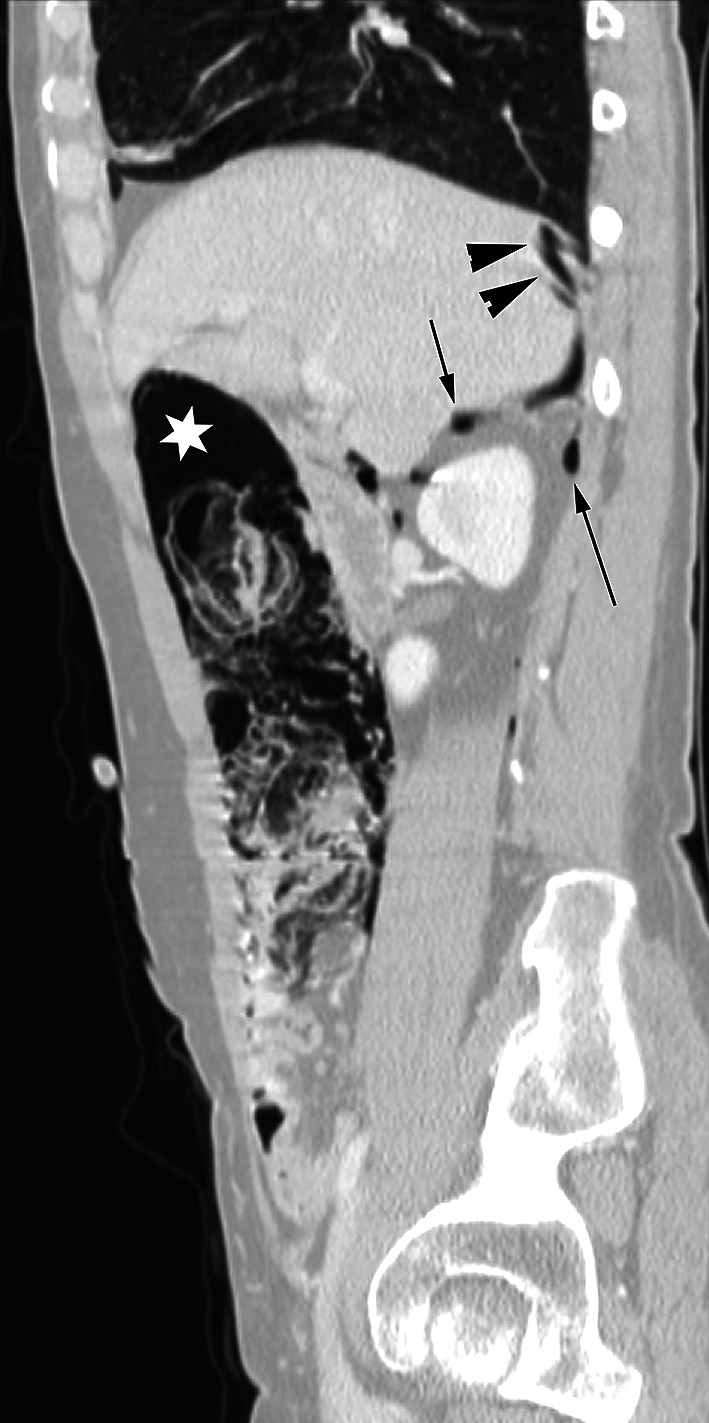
Extraperitoneal free air. Sagittal CT image shows pneumatosis of the ascending colon and extraluminal air in the adjacent mesentery (*asterisk*). This extraperitoneal free air tracks in the anterior and posterior pararenal spaces (*arrows*) to the bare area of the liver and diaphragm (*arrowheads*).
